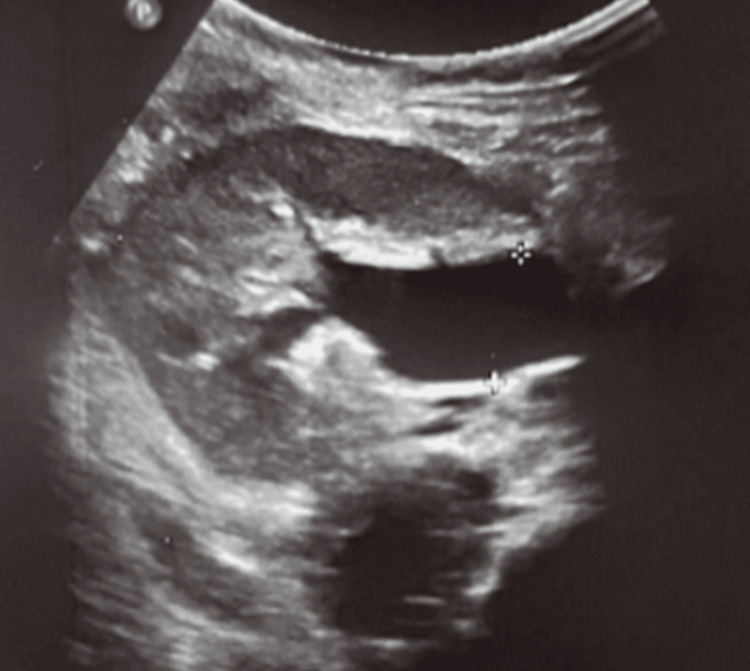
Ultrasound at four months post-transplant demonstrating persistent hydronephrosis (1.9 cm) without clear ureteral dilation
